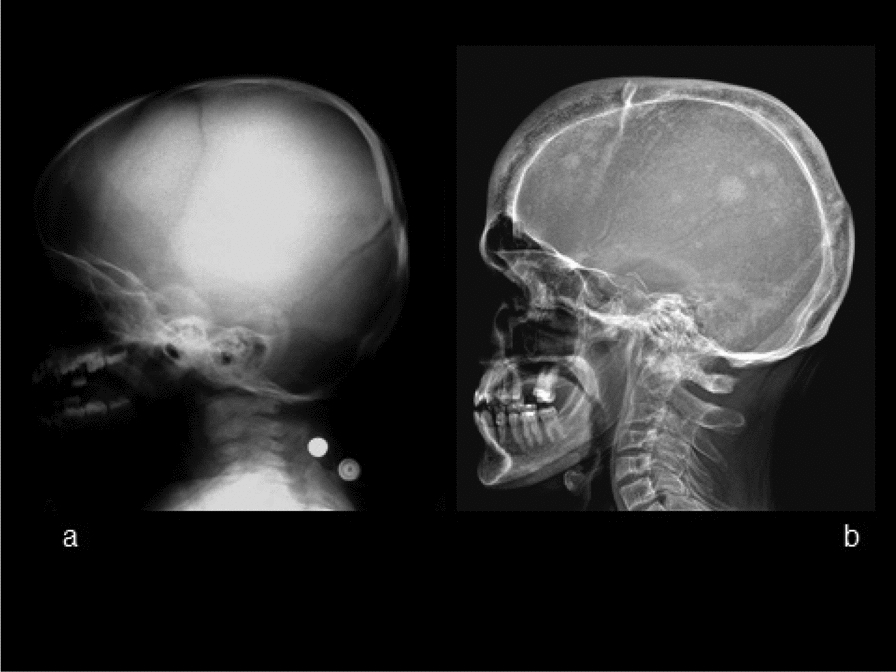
Lateral radiography of the skull and a part of cervical spine (a: age 1Y; b: age 21Y). The radiograph in infancy reveals diffuse osteosclerosis of the skull—both of the calvarium and of the base, without a prominent widening yet. The radiograph in early adulthood reveals marked widening of the skull with periosteal appositions both on the internal and external lamina, bossing of the frontal sinus, diffuse osteosclerosis as well as „cotton-wool “lesions of focal osteosclerosis. Discrete compressions of C3–C5 vertebral bodies are noticeable in the partially shown cervical spine. The vertebral body of C6 has the structure of coarse vertical trabeculae slightly framed by a thickened cortical bone (the image of a „framed vertebra”)
